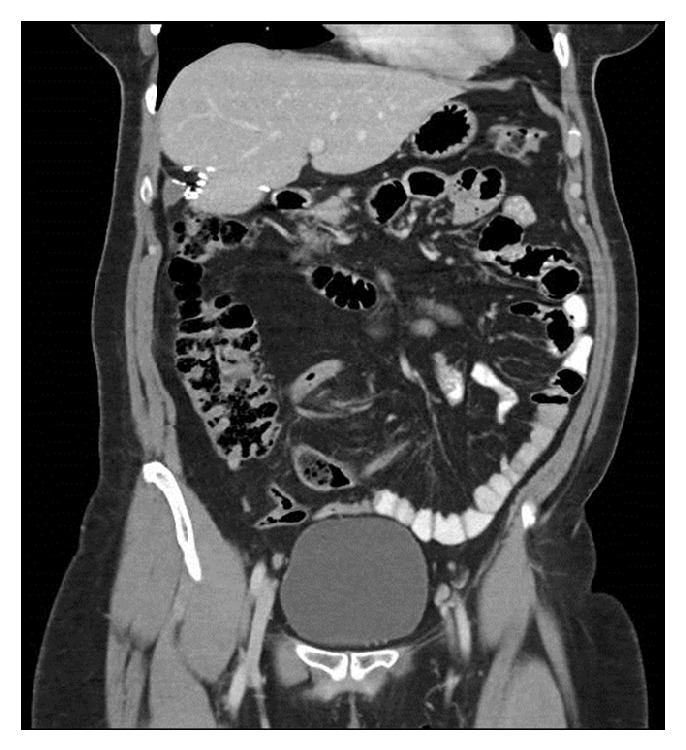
Coronal view of follow-up CT scan showing resection margin and no evidence of recurrence.
